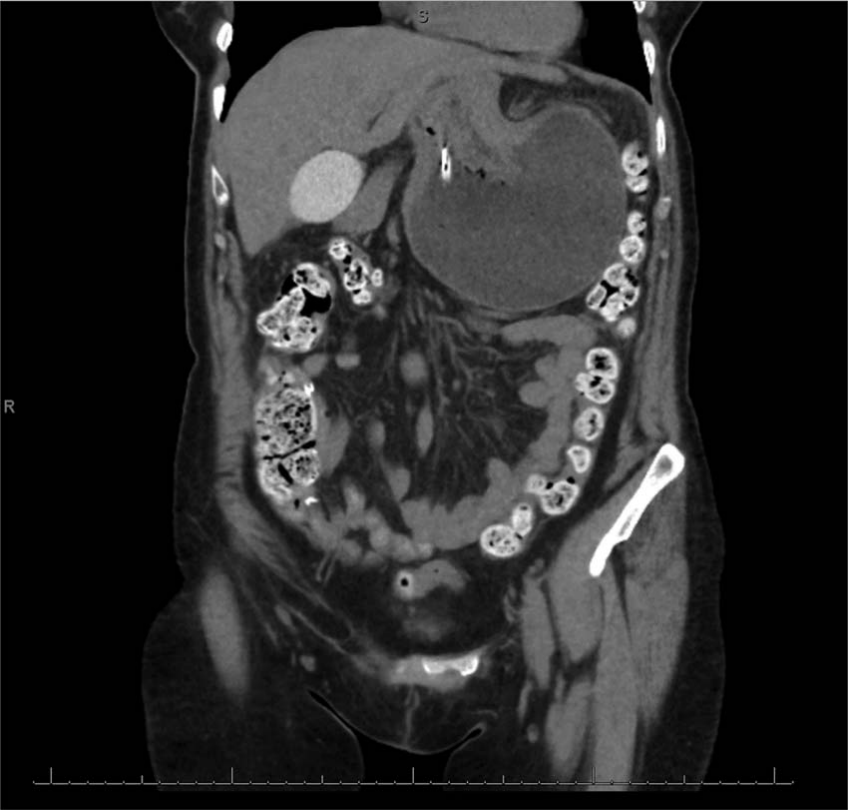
Coronal view of CT scan of abdomen and pelvis demonstrating gastric volvulus.
